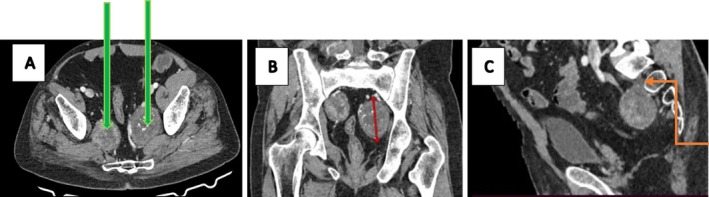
Contrasted CT scan of the pelvis, axial slice (A), coronal (B), and sagittal reformats (C) showing two well‐demarcated heterogeneously enhancing soft tissue‐density masses within the pelvis bilaterally, pre‐sacral in location with a connection to the anterior sacral neuroforamen (orange step arrow in sagittal view).
